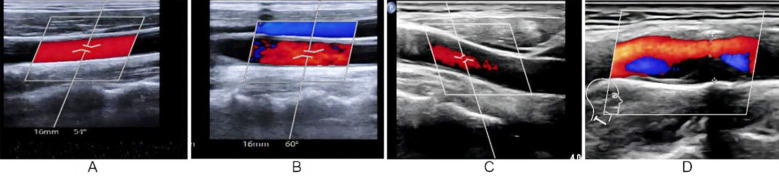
Neck vascular ultrasound of patients with different infarct sizes. A: Color Doppler ultrasound of normal and healthy neck blood vessels, no stenosis observed; B: Mild stenosis of the neck blood vessels can be seen on color Doppler ultrasound in patients with small area cerebral infarction; C: Moderate stenosis of the neck blood vessels can be seen on color Doppler ultrasound in patients with moderate area cerebral infarction; D: Severe stenosis of the neck blood vessels can be seen on color Doppler ultrasound in patients with extensive cerebral infarction.
